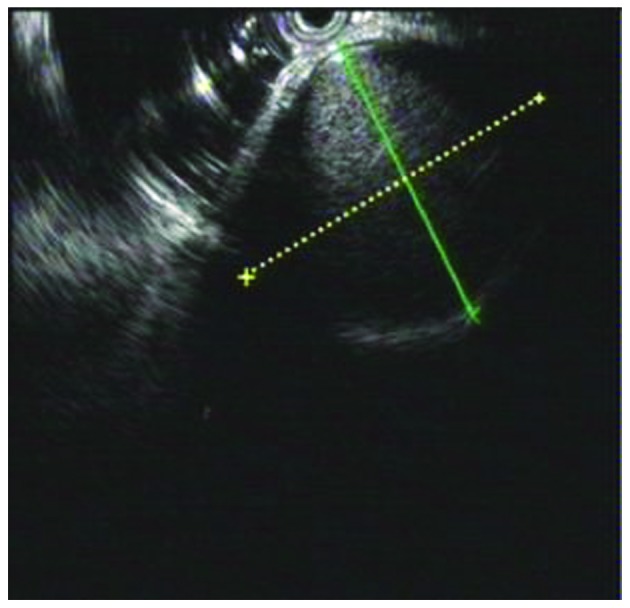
Endoscopic ultrasonography revealed a tumor with low homogenous echogenicity originating in the gastric muscular layer.
